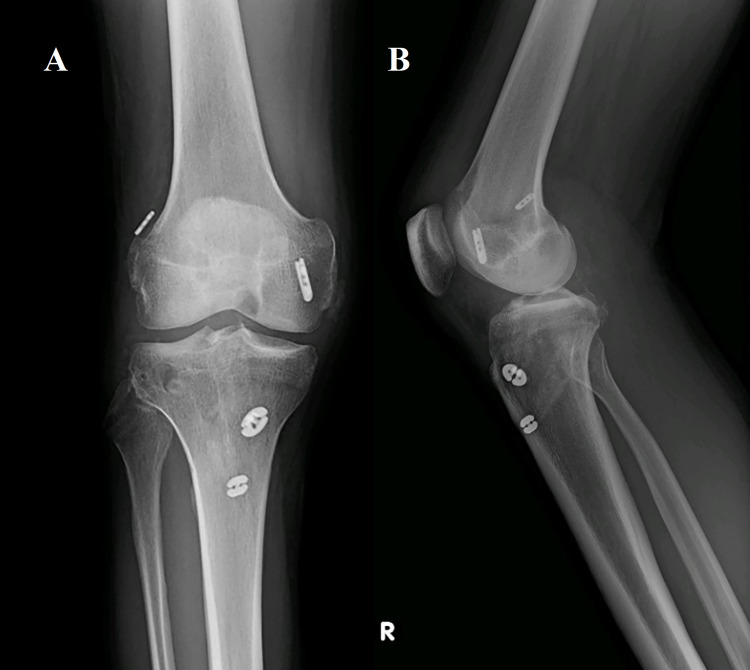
Right knee postoperative radiographs. Final right knee postoperative radiographs in the anterior-posterior (A) and lateral views (B).
